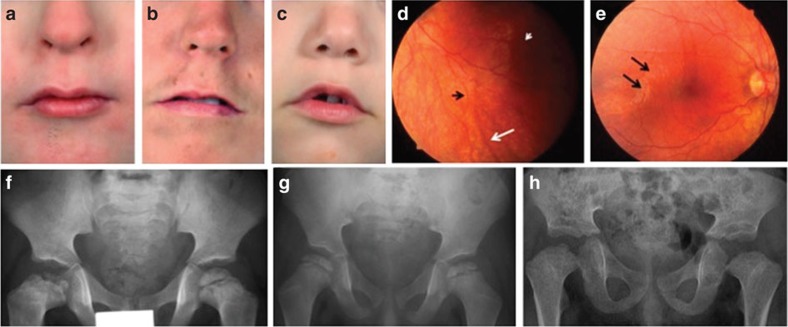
Facial, retinal and skeletal features of Roifman Syndrome. Facial dysmorphic features (a–c) include a markedly long philtrum, thin upper lip and down-turned corners of the mouth. Retinal features are displayed for patient 3 at age 4 years: arteriolar attenuation (d, black arrow), wrinkling of the inner limiting membrane (d, short white arrow and e, black arrows) and pigmentary changes (d, long white arrow). Skeletal features are displayed for patients 1, 3 and 5, respectively: the radiographs show the proximal epiphyses of the femora with symmetric delayed ossification, as well as flattening and irregularity.
